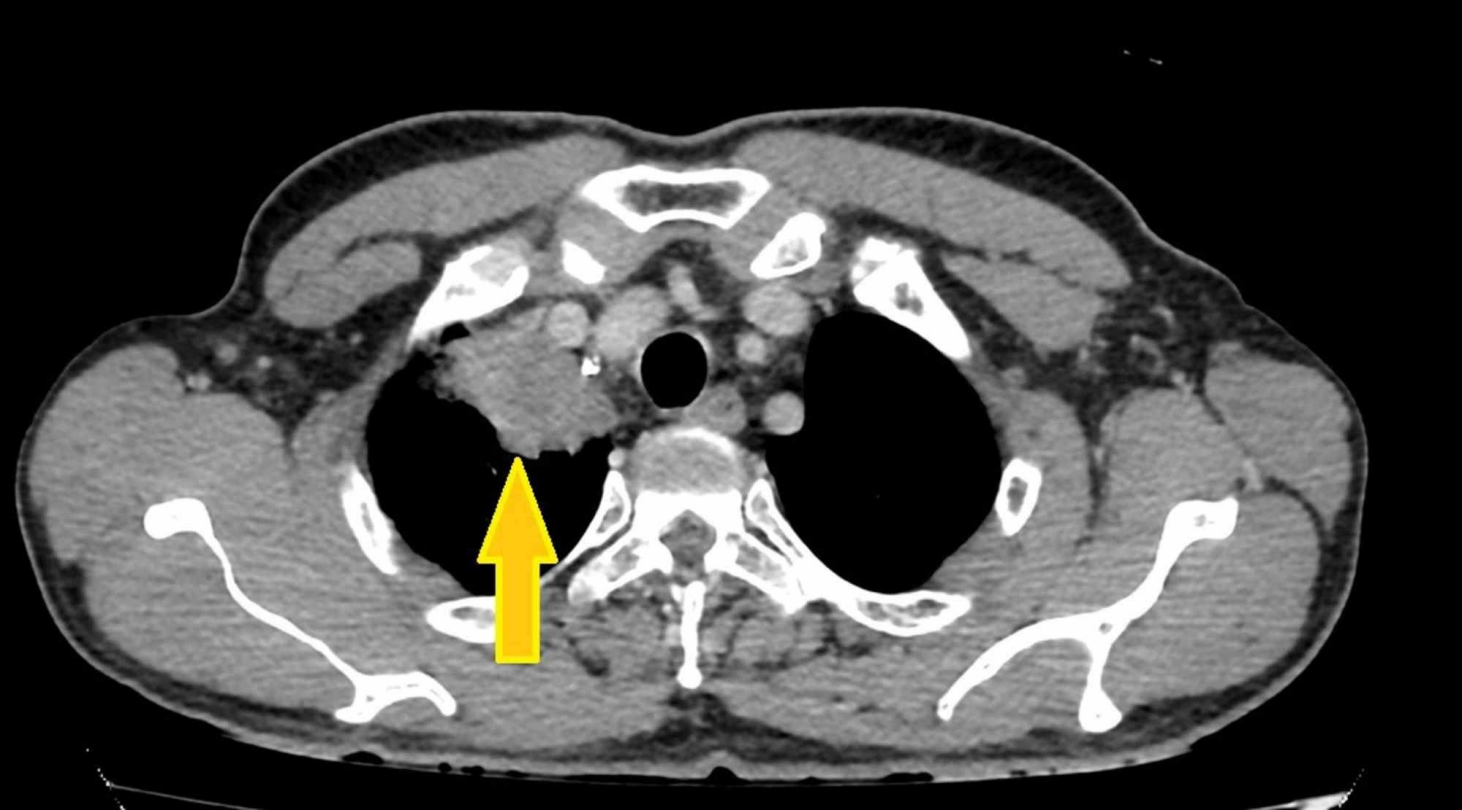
CT showing the lesion compressing adjacent structures. CT is the modality of choice for this malignant presentation [9].
